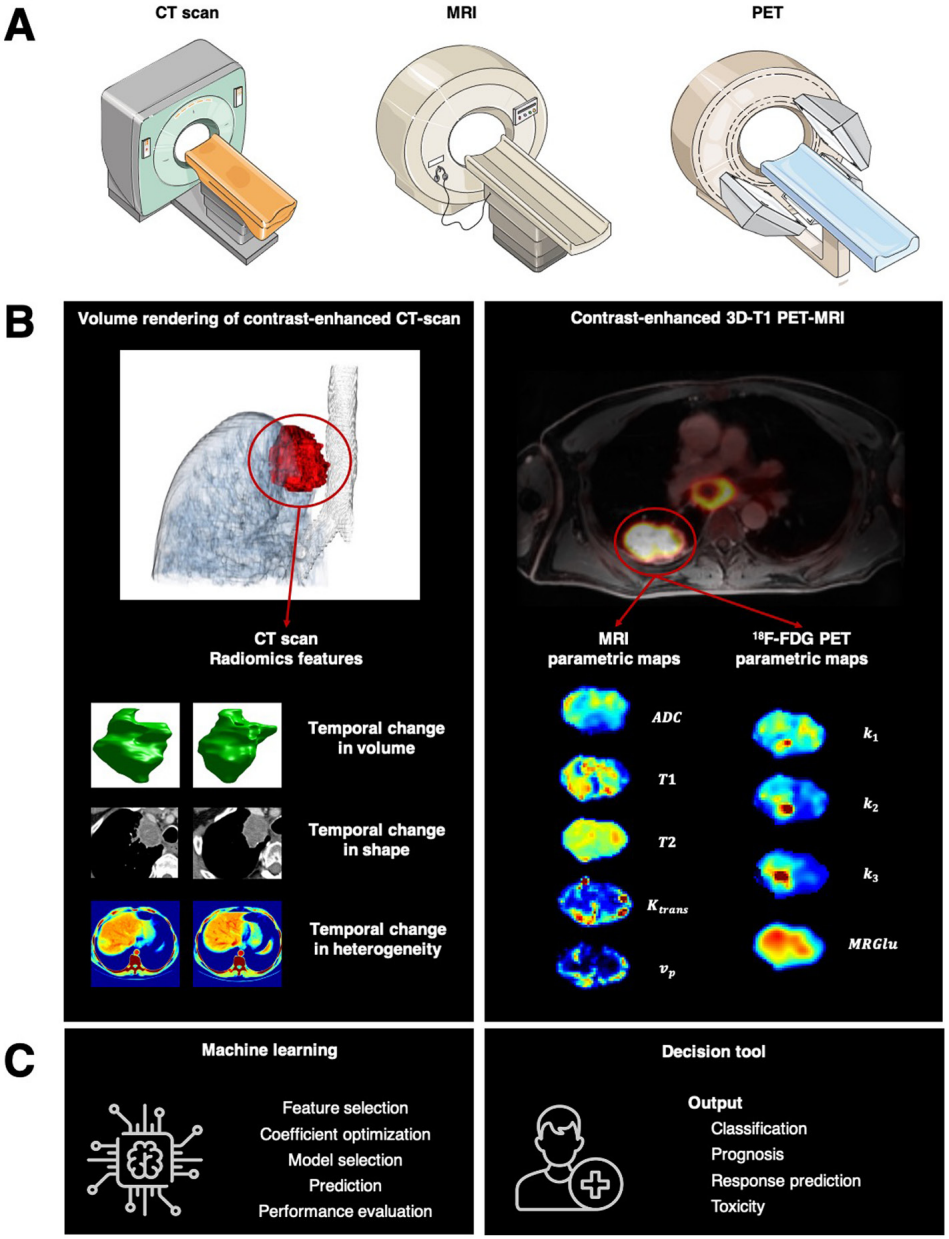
Toward radiomics for assessment of response to systemic therapies in lung cancer. (A) Multiple imaging modalities can characterize tumor imaging phenotypes such as CT scan, MRI, and PET. Multiparametric imaging offers unique opportunities to extend the image-based tumor analyses to more holistic characteristics. (B) left. In “Identification of Non-Small Cell Lung Cancer Sensitive to Systemic Cancer Therapies Using Radiomics,” the authors demonstrated that change over serial radiographic measurements in radiomics features deciphering tumor volume, invasion of tumor boundaries, or spatial tumor heterogeneity predicted tumor sensitivity to treatment, offering an approach that could enhance clinical decision-making to continue systemic therapies and forecast overall survival. (B) right. A fundamental breakthrough would be integrating multiparametric imaging data into the radiomic framework, such as imaging features extracted from functional MRI and 18F-FDG PET. (C) Machine-learning approaches can unravel among these imaging features, new imaging biomarkers predicting tumor sensitivity to treatment.
